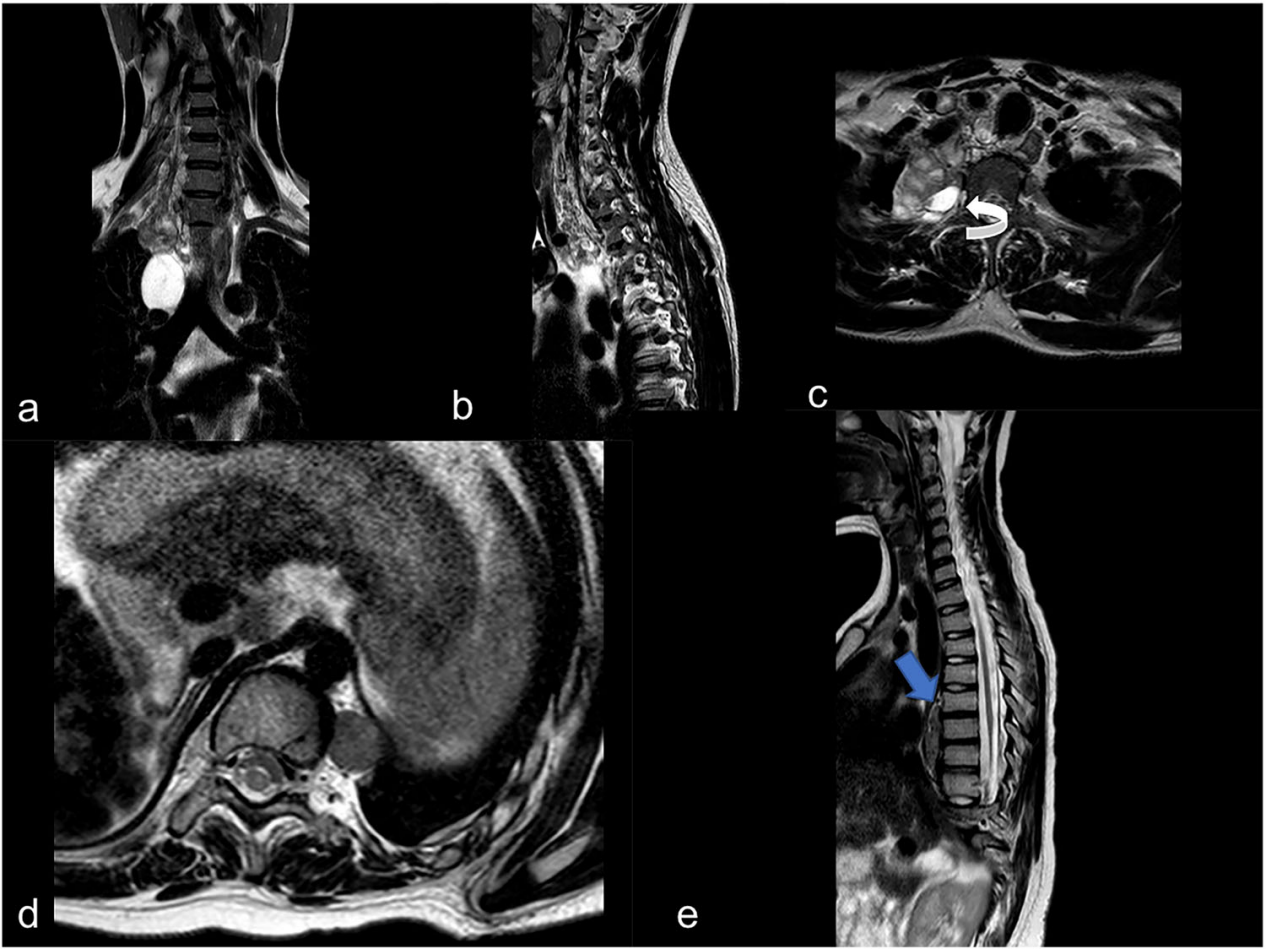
MRI-T2 sequences with fat suppression. a–c Neurofibroma arising from the neck and extending to the upper thoracic site, (c) widened right neural exit foramina (white curved arrow). d, e A typical case of pediatric paravertebral neurinoma with a rounded aspect (d). In this case, lesions do not cause bone erosion but vertebral scalloping in the thoracic vertebral body at the level of Th5 (blue arrow). Both tumors show shade hyperintense aspects on T2-weighted sequences
